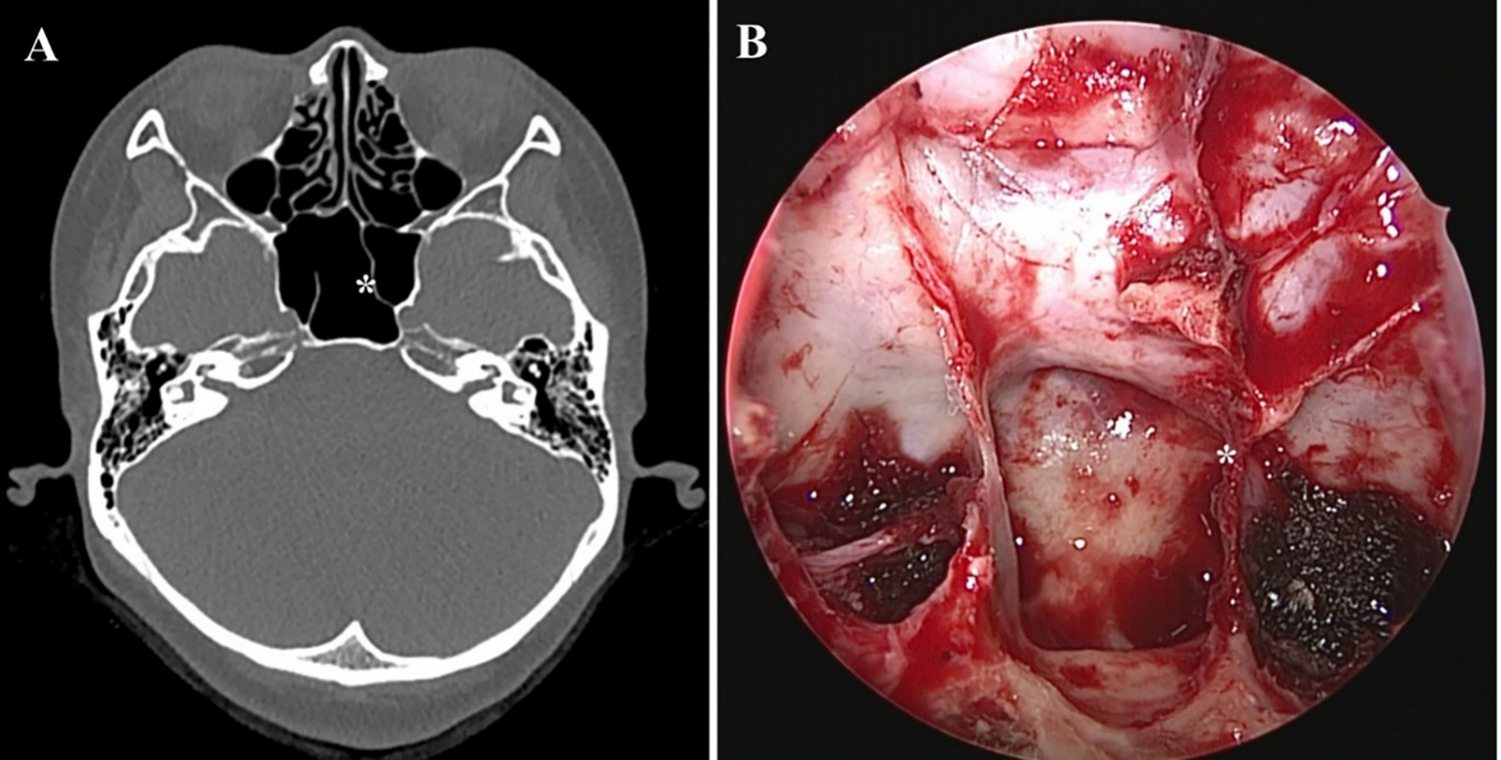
An intersphenoid sinus septum (asterisk) inserted into the paraclival internal carotid artery in a patient with postsellar pneumatization, as revealed by preoperative paranasal sinus computed tomography (A) and during endoscopic endonasal transsphenoidal surgery (B).
